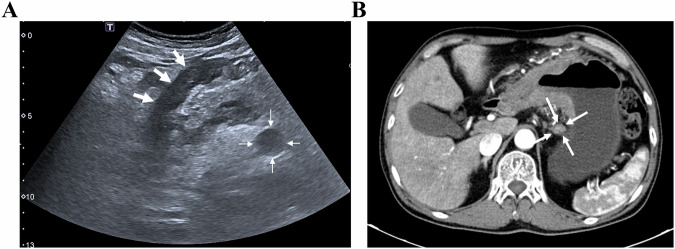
A An approx 60-year-old man underwent gastric oral contrast ultrasonography, revealing full-thickness thickening of the gastric wall of the gastric antrum (white thick arrow), multiple lymph node enlargement around (white thin arrow). B Multiple lymph node enlargement at the lesser curvature of the stomach (N3) on enhanced computed tomography
